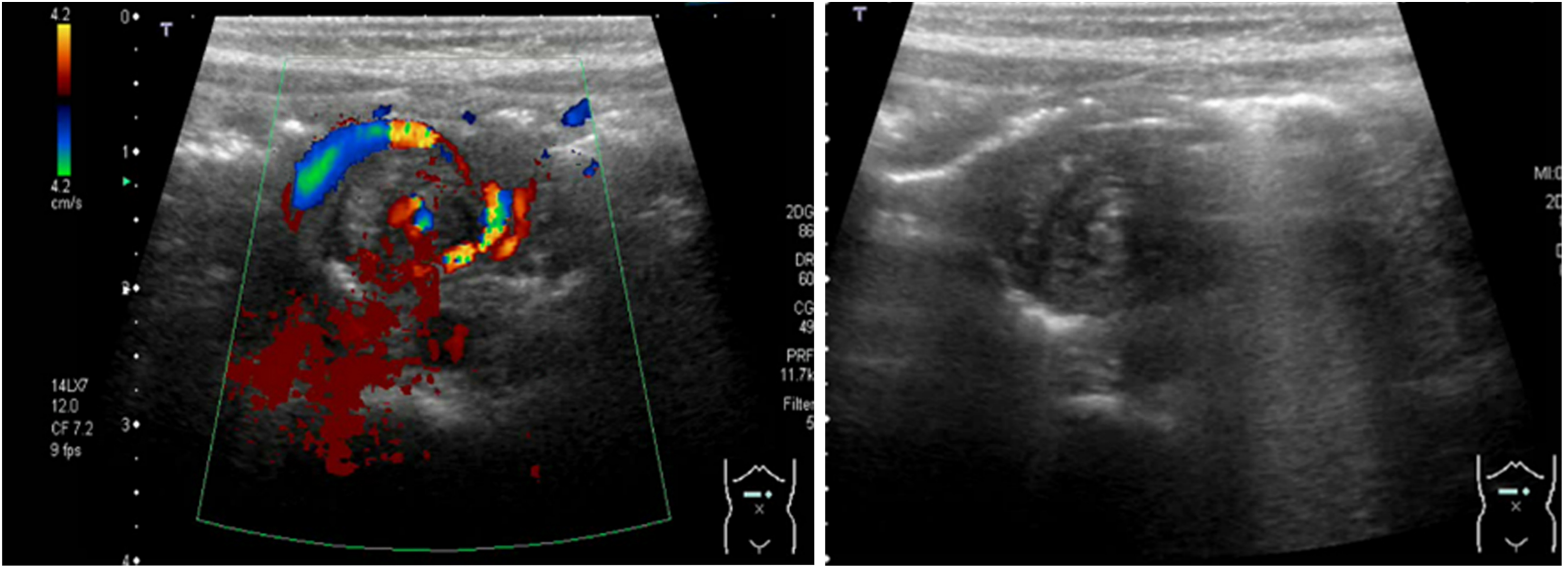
The sonographic appearance of neonatal midgut volvulus—the whirlpool sign. Color Doppler. Grayscale imaging.
